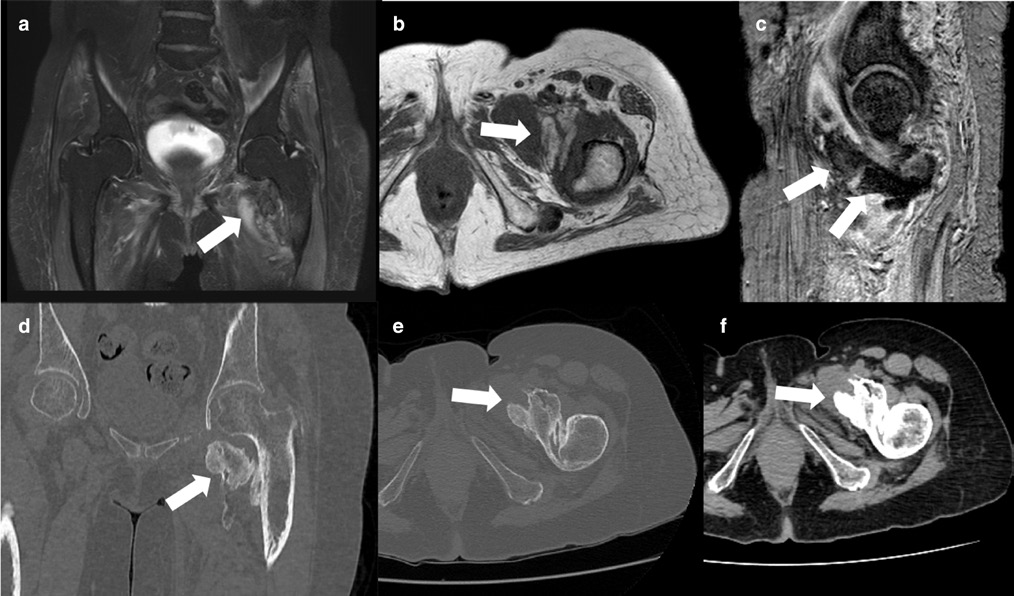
Hip MR. (A) Coronal T2 fat-saturated, and (B) axial T1 and (C) sagittal GRE imaging demonstrated anterior periarticular heterotopic ossification. Left hip CT coronal (D) axial (E) and axial soft-tissue window (F) images showing a large anterior periarticular heterotopic ossification. GRE, gradient-echo.
